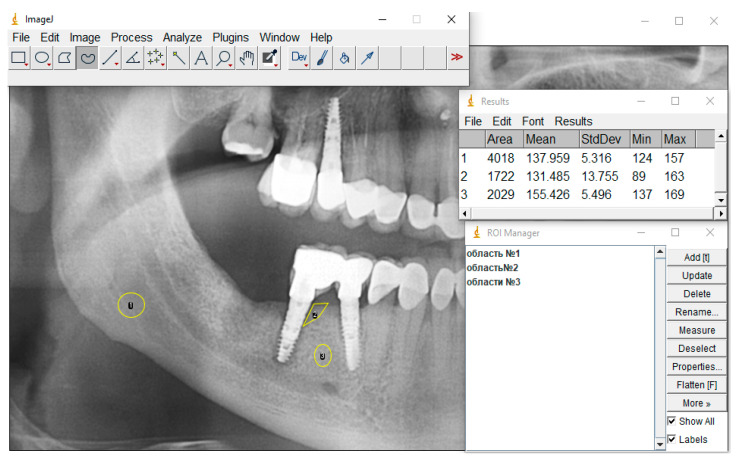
X-ray picture after treatment: where area 1 is the standard, area 2 is peri-implantitis, and area 3 is the area without signs of pathological changes in bone tissue.
